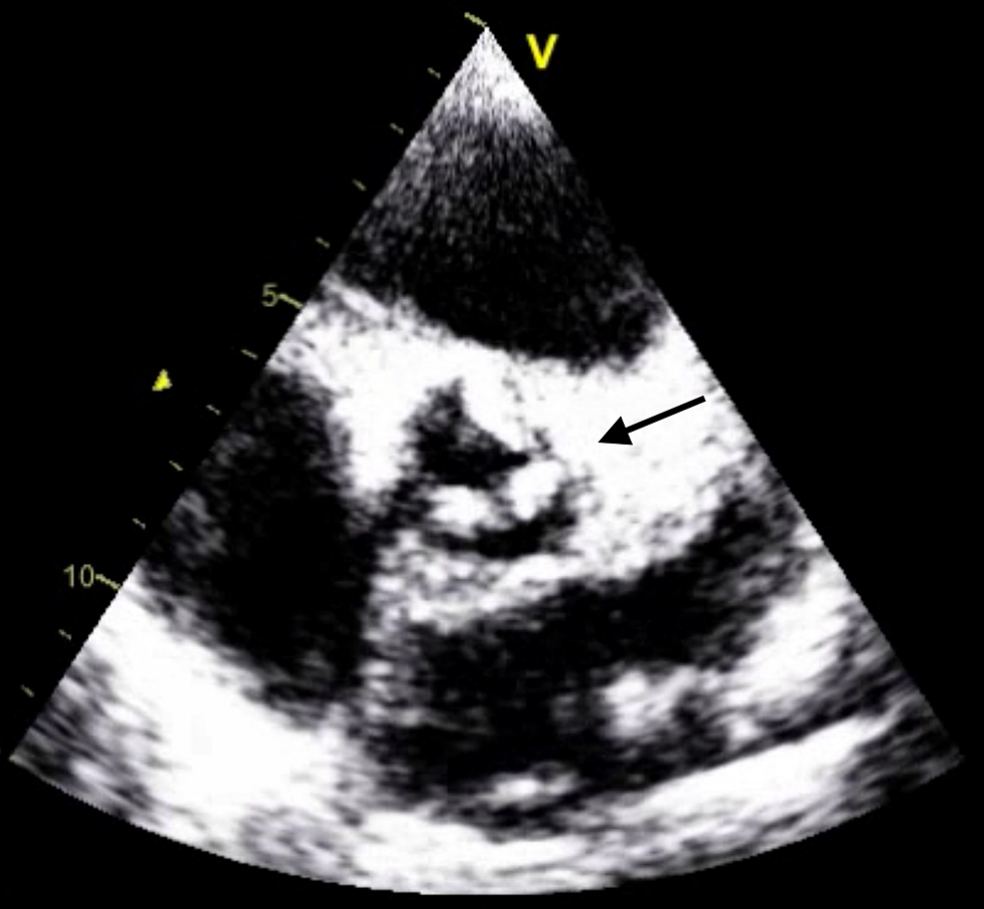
Large echogenic densities were noted on the aortic valve on the transthoracic echocardiogram.
